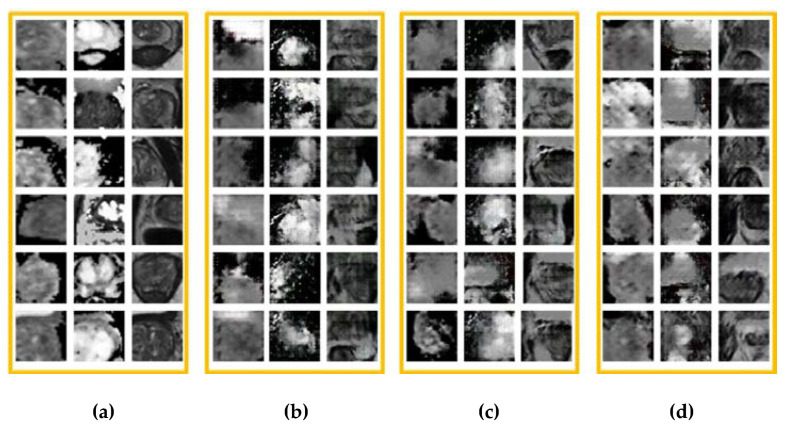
Comparison of real and generated MR images, where the first, second and third columns in each orange box are ADC images, DCE images and T2W images, respectively: (a) the real images, (b–d) are the generated images using the DCGAN, LSGAN, and CapGAN models, respectively.
